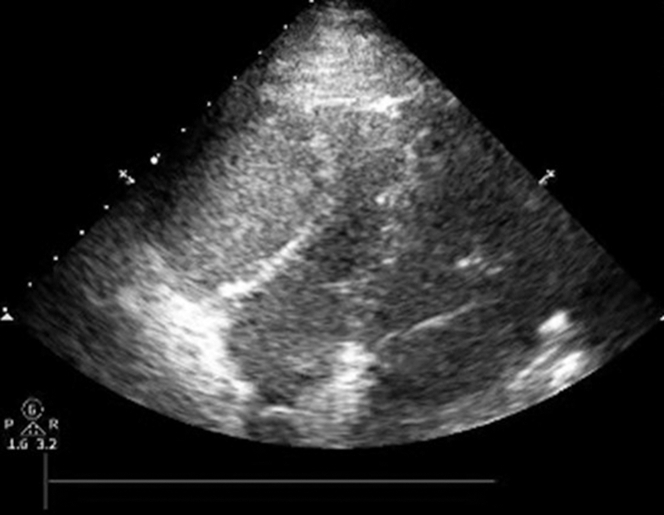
TTE Demonstrating Right Ventricular Compression TTE showing right ventricular compression concerning for tamponade. Subsequently, immediate epicardial drain was placed. TTE = transthoracic echocardiogram.
